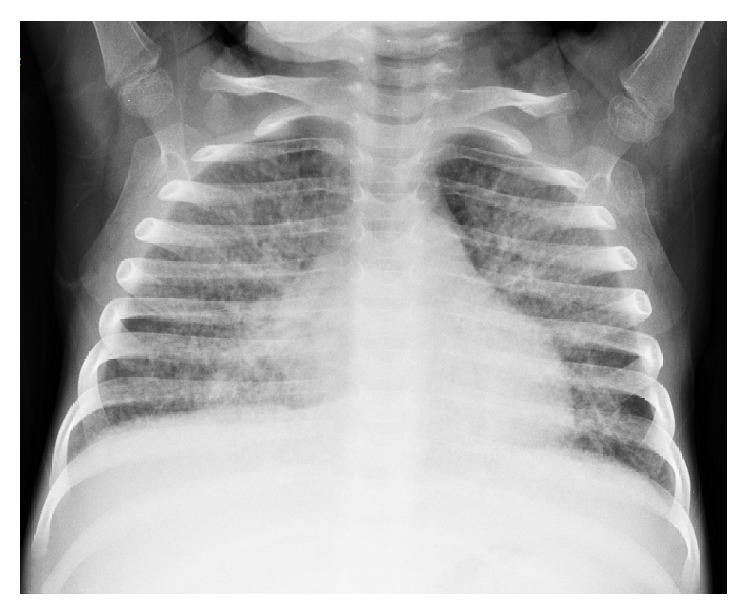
Bilateral lung interstitial infiltration in chest radiography.
